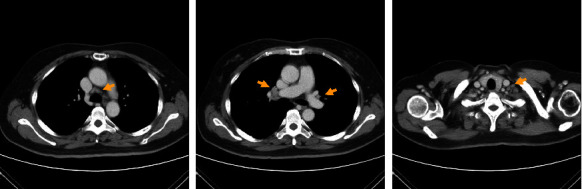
Chest computed tomography (CT) scan revealed borderline enlarged mediastinal, both hilar and left supraclavicular lymph nodes.
